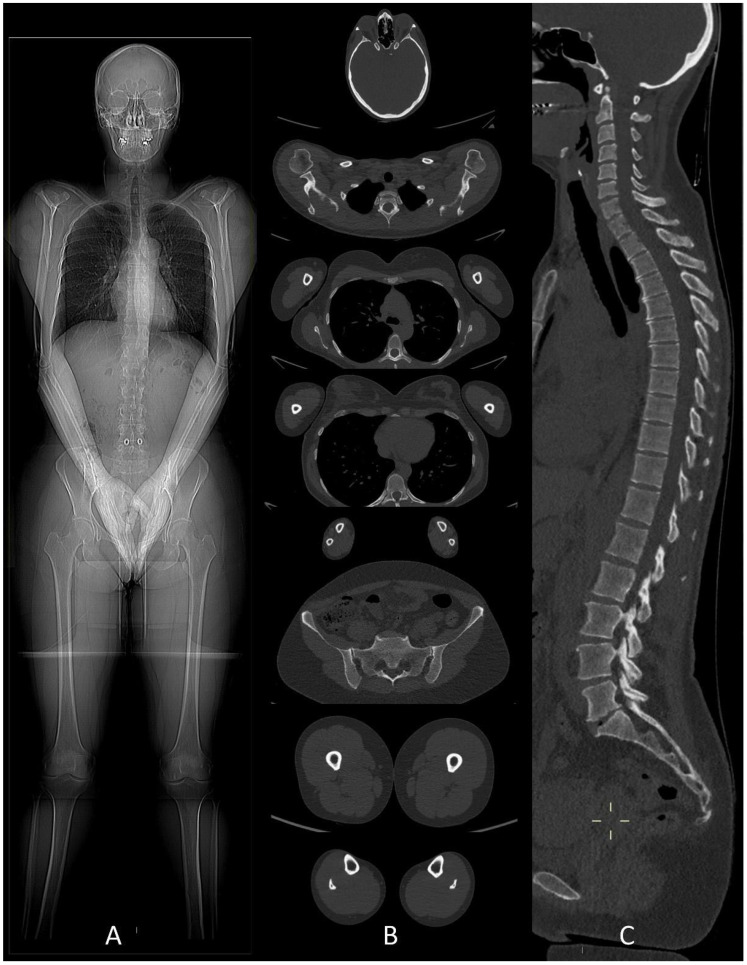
(A) CT scout-view showing the correct patient position in the CT scanner to avoid beam-hardening artifacts on thoracic and lumbar spine. (B) Whole-body low-dose CT examination performed from the skull down to the lower limbs. (C) Sagittal CT image showing cervical, dorsal and lumbar spine with “bone” algorithm is the best plane to identify vertebral compression fractures.
